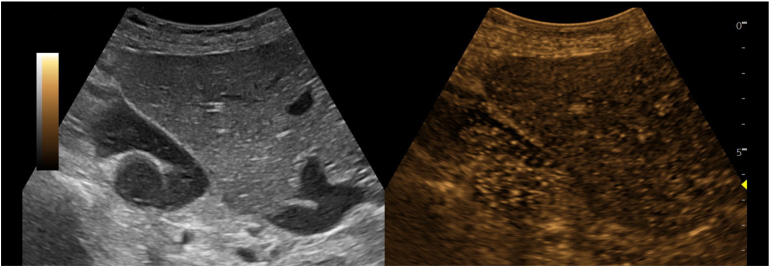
Intraoperative contrast-enhanced ultrasound. Following the intravenous administration of a 1.6 mL bolus of ultrasound contrast agent (SonoVue, Bracco Imaging S.p.A.), the liver demonstrated homogeneous parenchymal enhancement across the entire organ with no perfusion deficits. Furthermore, no focal lesions, regions of portal-venous washout, or signs of thrombosis were evident. Split-screen images are presented with contrast-enhanced ultrasound 80 seconds after injection of the contrast agent on the right and simultaneous corresponding B-mode gray-scale imaging on the left.
